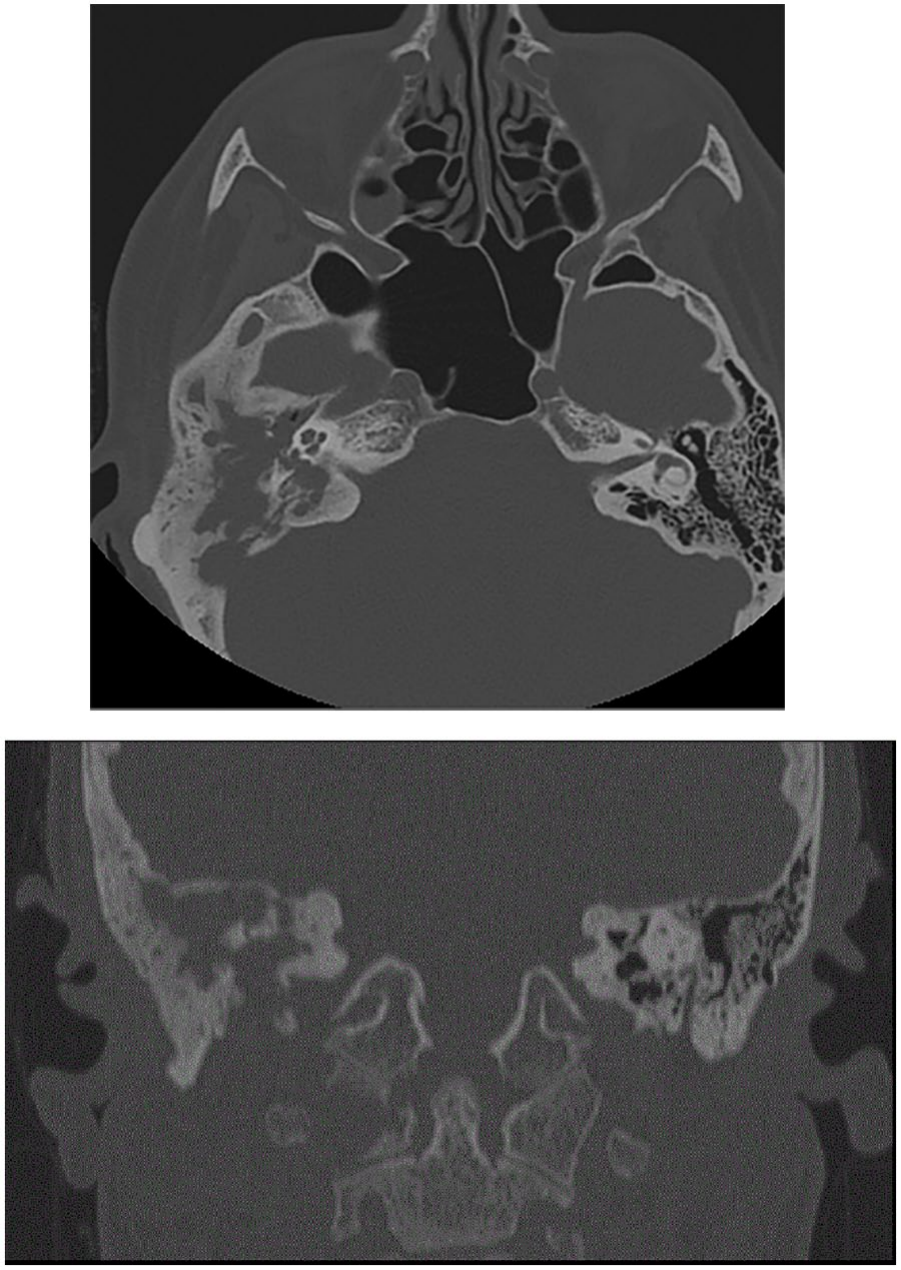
Axial and coronal computed tomography image shows opacification and calescent destructive changes of right mastoid air cells, erosions of right tegmen, middle ear ossicles, and sigmoid plate as well as osteolytic lesion in right lateral mass of C1.
